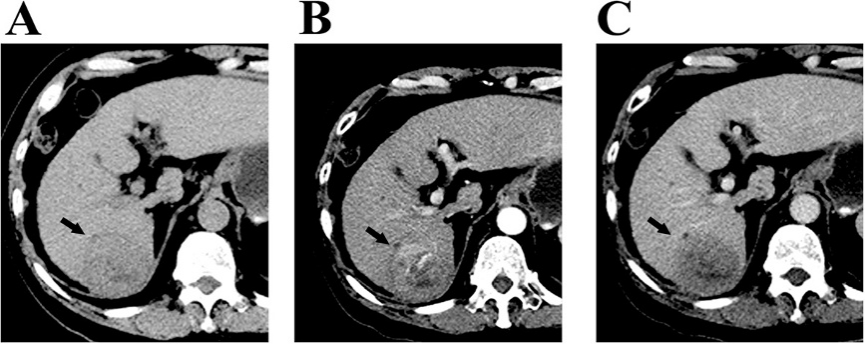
Contrast-enhanced CT images obtained in a patient with 6-cm single HCC before TACE treatment. (A-C) show a patient with hepatitis B-induced liver cirrhosis and a 6-cm solitary HCC tumor in the hepatic segment VI. The contrast-enhanced CT scan before TACE revealed arterial enhancement of the HCC lesion.
